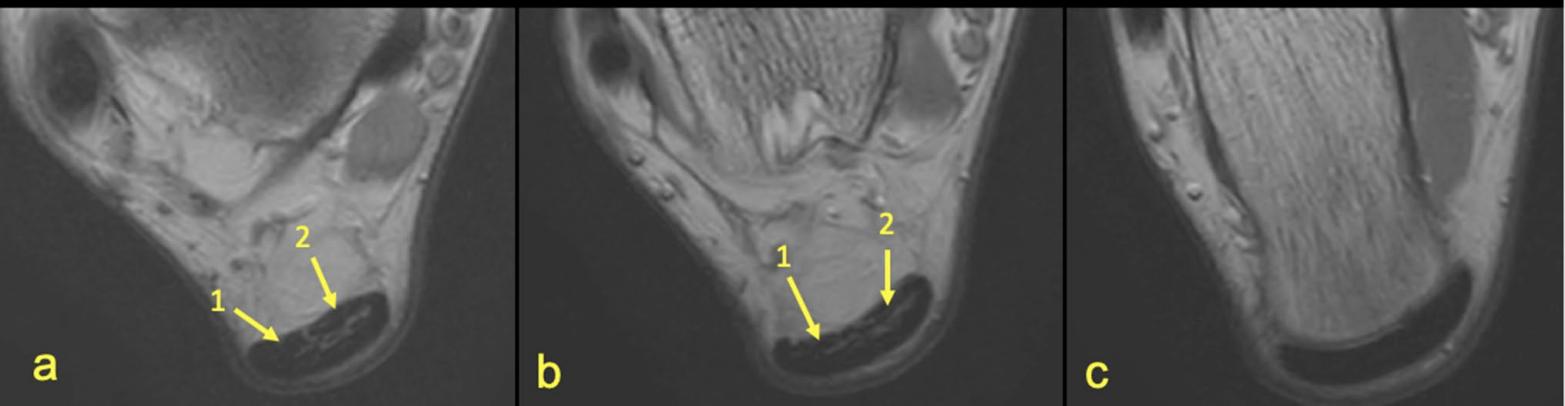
MRI right ankle, PD FS at the level of the talus (a), superiorly to the calcaneus (b) and the Achilles tendon insertion (c). A 23-year-old male with a suspected anterior talofibular ligament rupture. 1—S-LGC, 2—S-Sol. It is not possible to clearly distinguish S-MGC.
